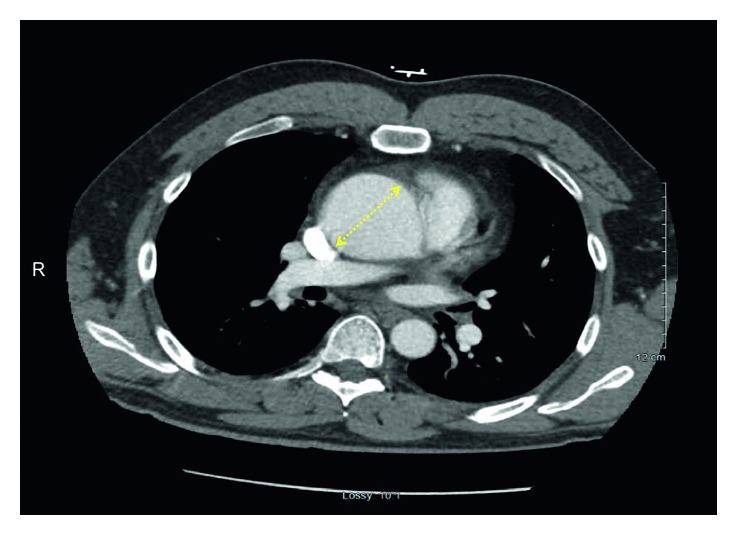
CT chest with contrast showing 6 cm aortic root (yellow dotted line).
